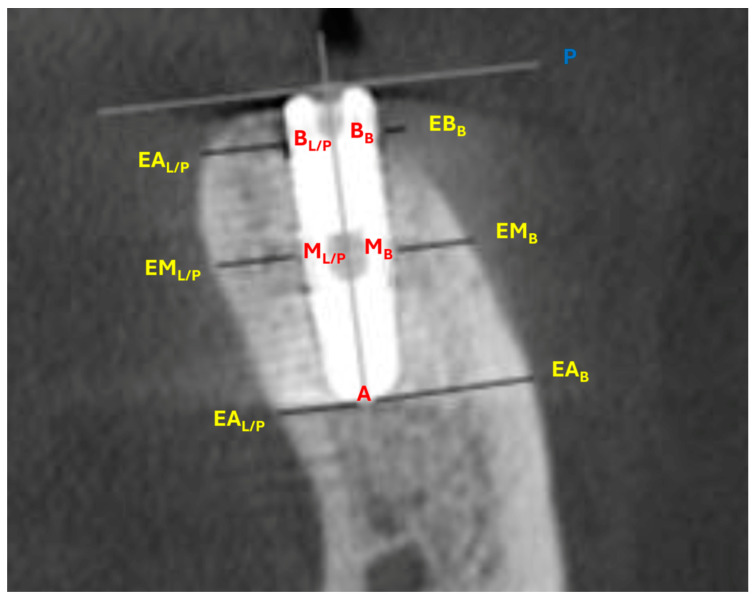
CBCT landmarks and measurements. Measurements were taken perpendicular to the implant vertical axis at the implant bevel plane. Palatal/lingual apex: distance from the most apical spire to the corresponding palatal/lingual external cortical bone landmark (A–EA_L/P_). Palatal/lingual medial: distance from the medial spire to the corresponding palatal/lingual cortical external bone landmark (M_L/P_–EM_L/P_). Palatal/lingual base: distance from the first spire to the corresponding palatal/lingual external cortical bone landmark (B_L/P_–EB_L/P_). Vestibular apex: distance from the most apical spire to the corresponding external buccal bone landmark (A–EA_B_). Vestibular medial: distance from the medial spire to the corresponding external buccal bone landmark (M_B_–EM_B_). Vestibular base: distance from the first spire to the corresponding external buccal bone landmark (B_B_–EB_L/P_). Vestibular gap: distance from the internal buccal bone landmark of the bevel/marginal spire to implant bevel plane (B_B_–P).
